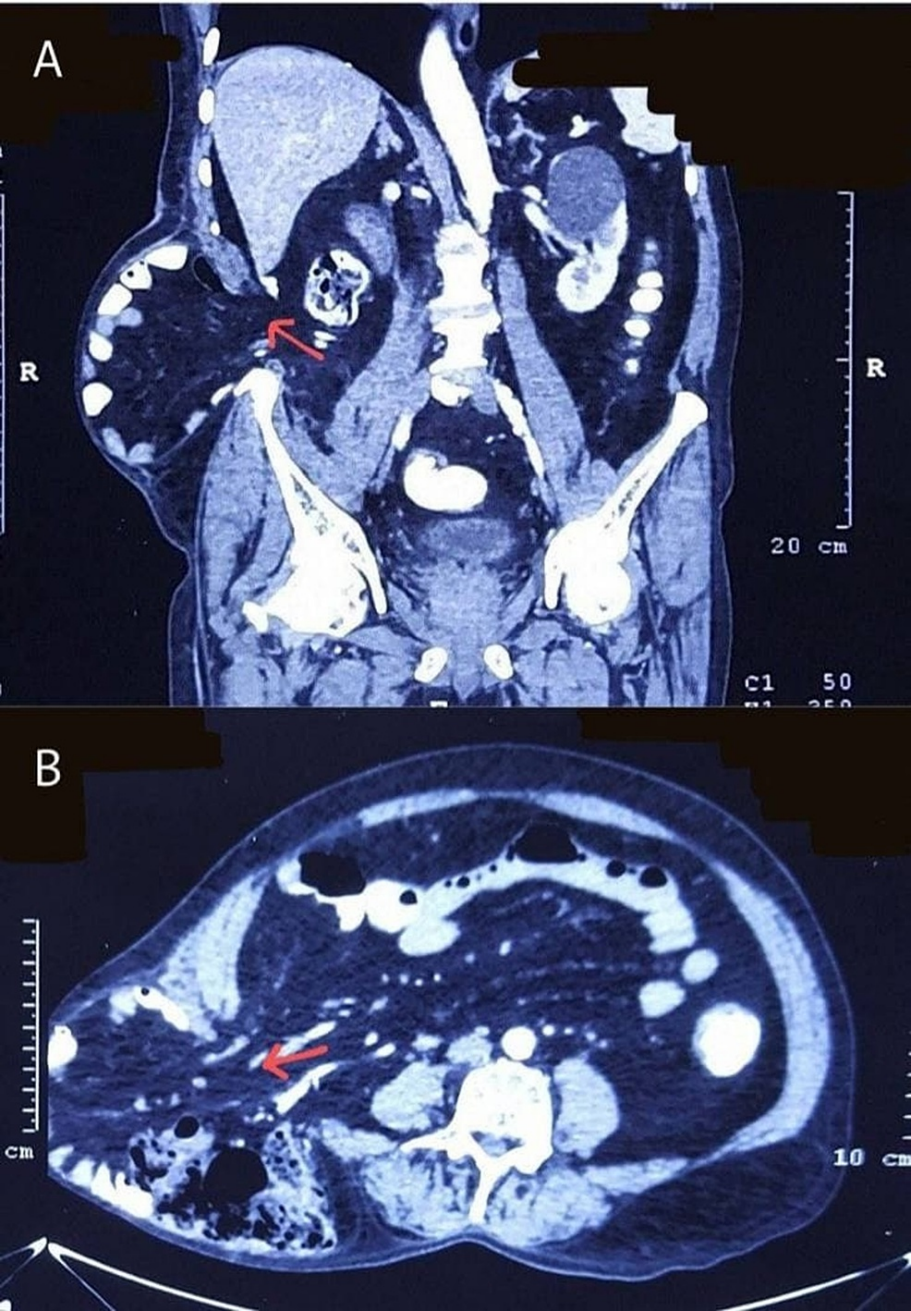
CECT abdomen with arrows pointing toward the defect and bowel loops seen herniating through the defect. (A) sagittal section. (B) Transverse section. CECT: contrast-enhanced computed tomography
